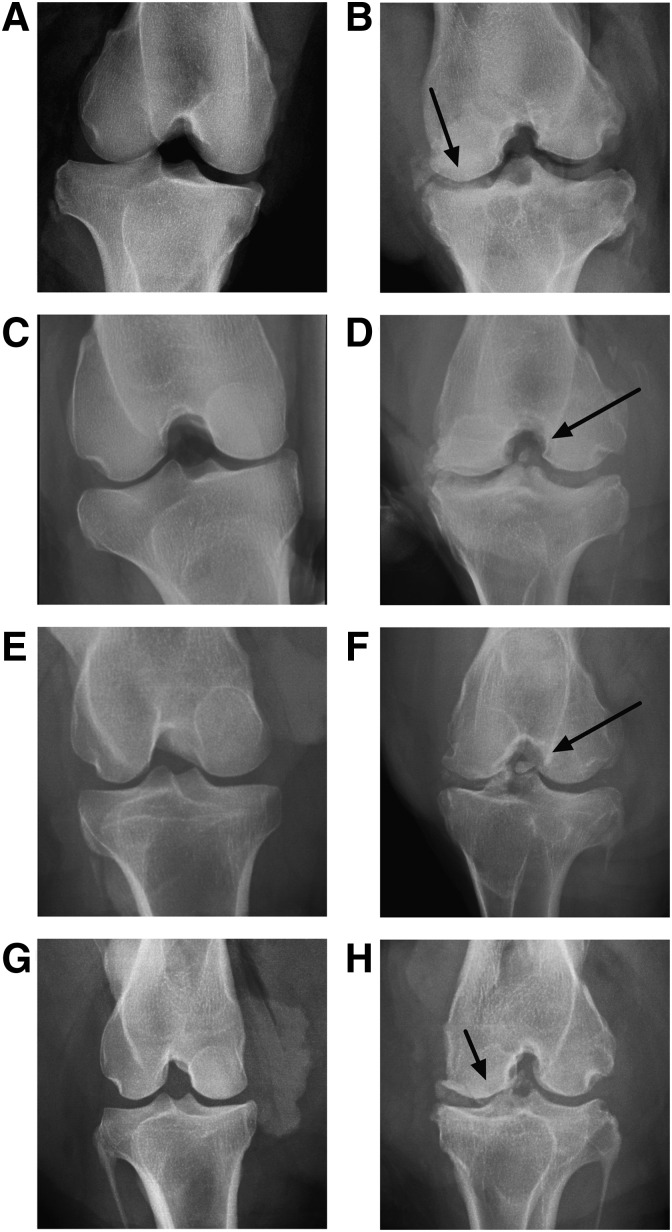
Explant radiographs. Anteroposterior views of contralateral and graft knees from BLB graft explants after 2-year implantation using fresh (B, D) and frozen (F, H) BLB constructs and associated contralateral knee (A, C, E, G). Arrows indicate loose bone fragments and osteophyte formations. BLB, bone–ligament–bone.
